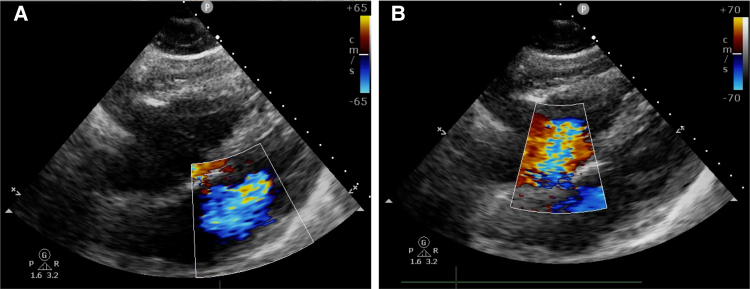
When attempting to extubate, the patient developed hypotension and hypoxaemia, with transthoracic echocardiography showing severe mitral and tricuspid regurgitation (A and B)
